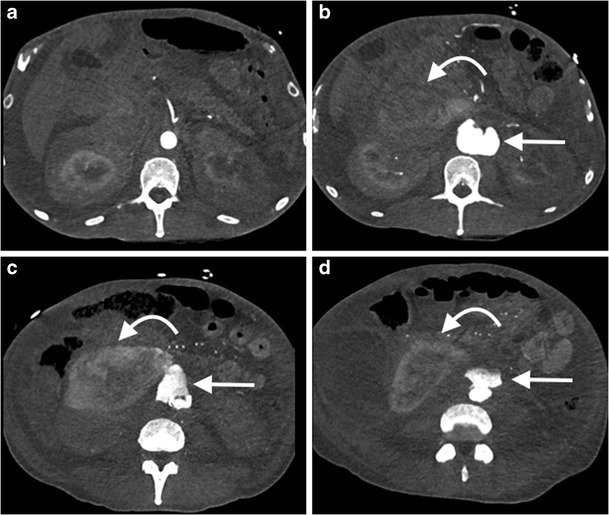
Selected axial images (a-d) from an arterial phase CT angiogram of the abdominal aorta in a 55-year-old man with hypotension and abdominal pain demonstrates a large retroperitoneal haematoma (*curved arrow*) and active extravasation from the infrarenal abdominal aorta (*arrow*) consistent with aortic rupture. A ruptured mycotic aneurysm was found in the operating room
